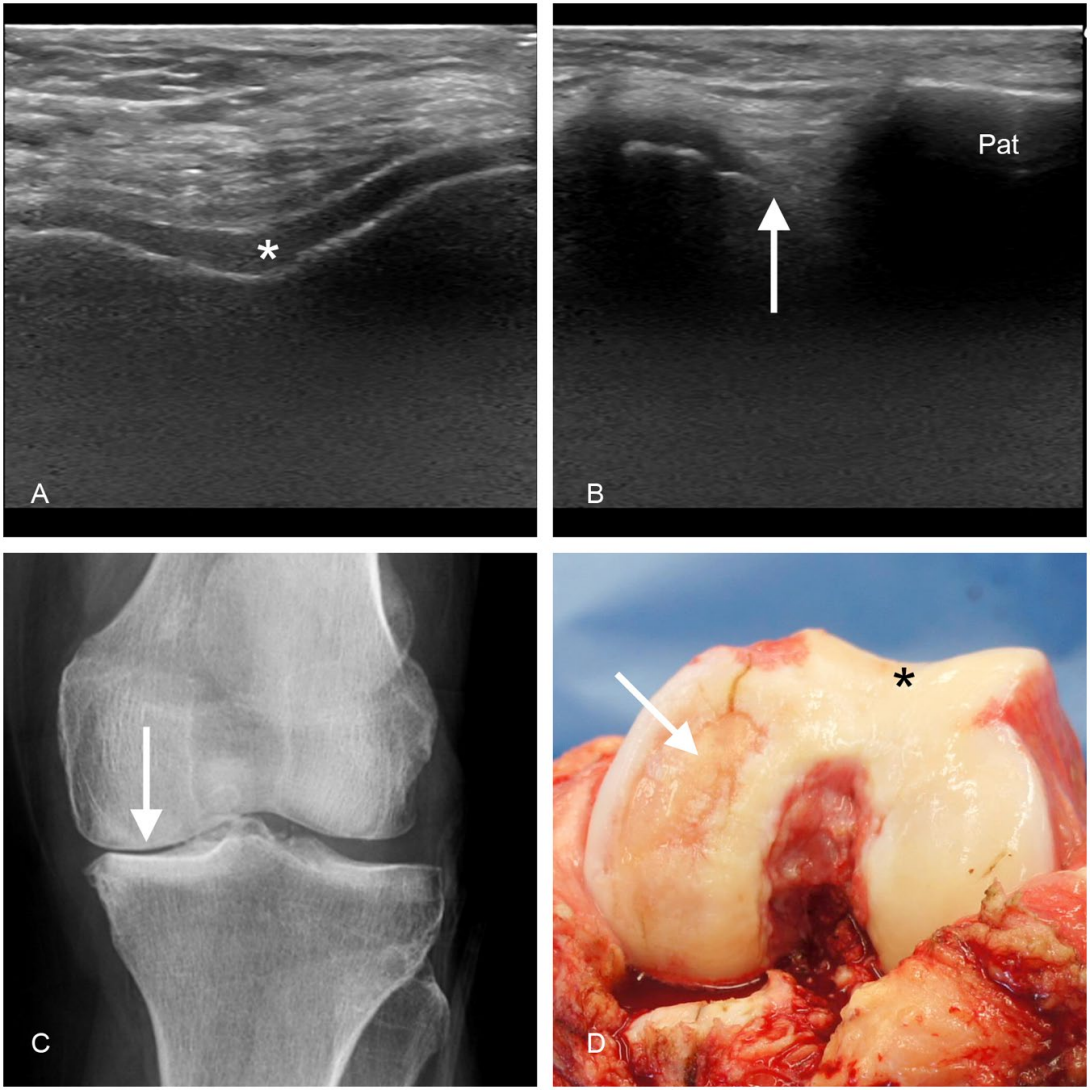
The cartilage view on ultrasonography (US), on radiography and during total knee arthroplasty (TKA). On femoral sulcus, axial US-view demonstrates a normal cartilage (white asterisk) without damage (A). On medial femoral condyle, axial parapatellar US-view (patella on the right side, marked as Pat) shows a distinct damage (arrow) on the cartilage (B). The corresponding radiography depicts a clear narrowing (arrow) of the medial joint space (C). Ultimately, the photography of femoral cartilage surfaces taken during the TKA reveals intact femoral sulcus (asterisk) and full-thickness cartilage damage of the medial femoral condyle (arrow) (D).
